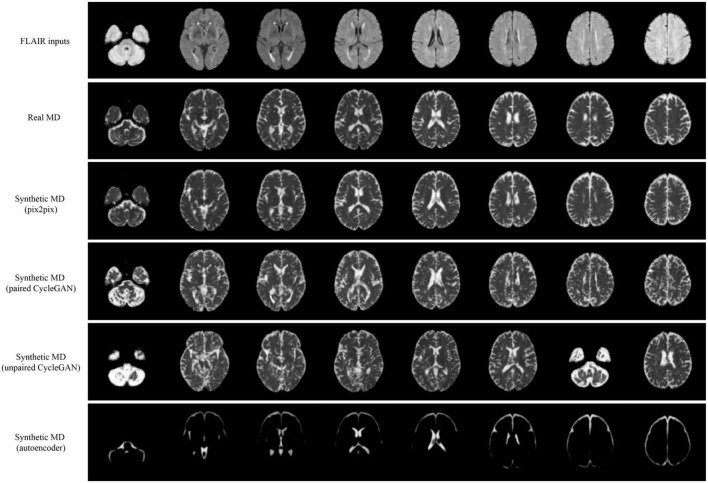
Samples of FLAIR inputs (top row), real MD (second row) and generated MD slices from each model. For paired models, the images shown are different slices from the same patient volume. From left to right, randomly sampled lower to upper slices (12 to 35) are shown.
